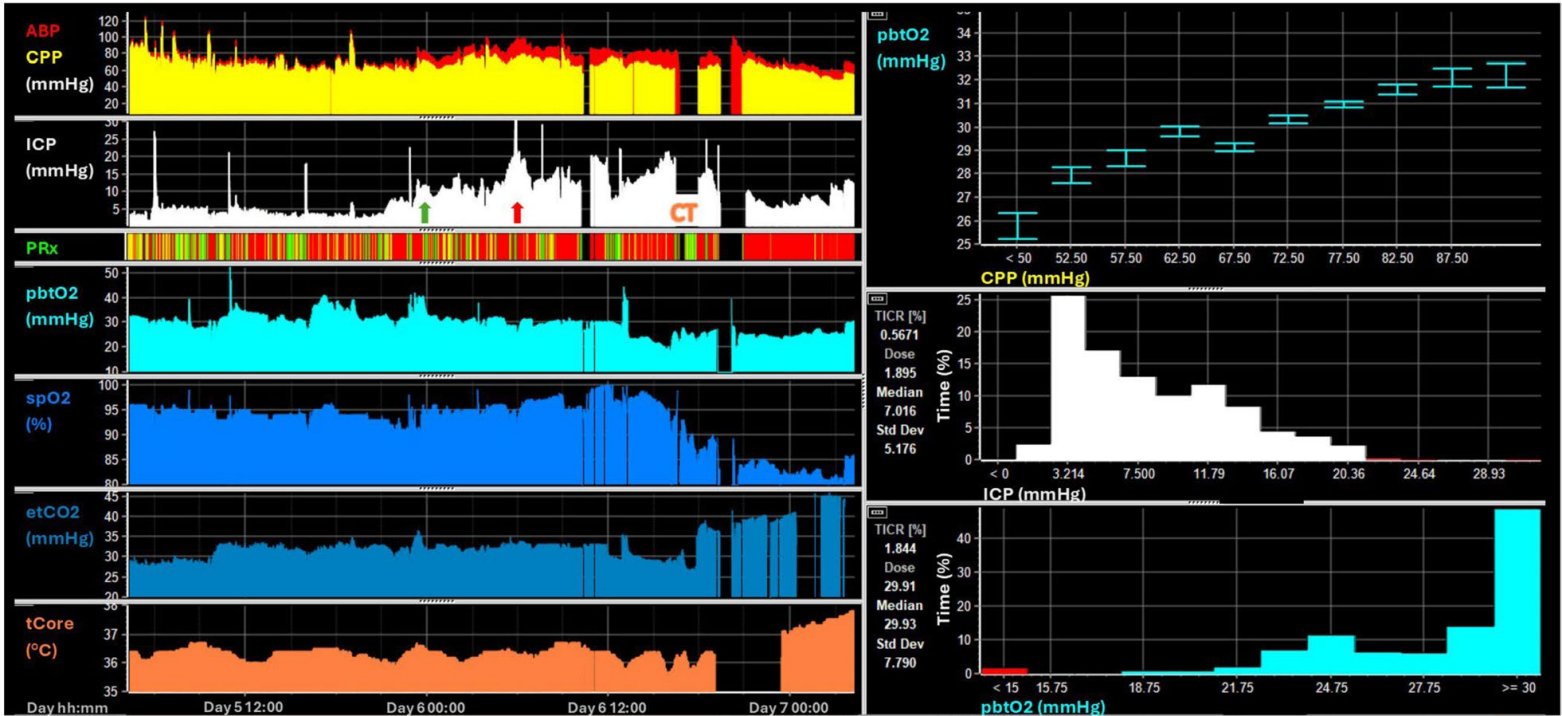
Multimodality data from case 2. The MMM data is displayed with minute-by-minute data on the left panels and derived doses and associations on the right. The green arrow displays the moment when sedation was reduced with an initial stable period of around 4 h before there was a secondary increase in ICP (marked with a red arrow). The distinct desaturation can be seen in the spO2 trace after the initiation of the thoracic CT. On the right top panel, the almost linear association between increasing CPP and PbtO2 can be seen. Below, the percentage time spent at the different ICP or PbtO2 levels are shown with only minimal time spent above an ICP of 20 mmHg and PbtO2 below 15 mmHg
